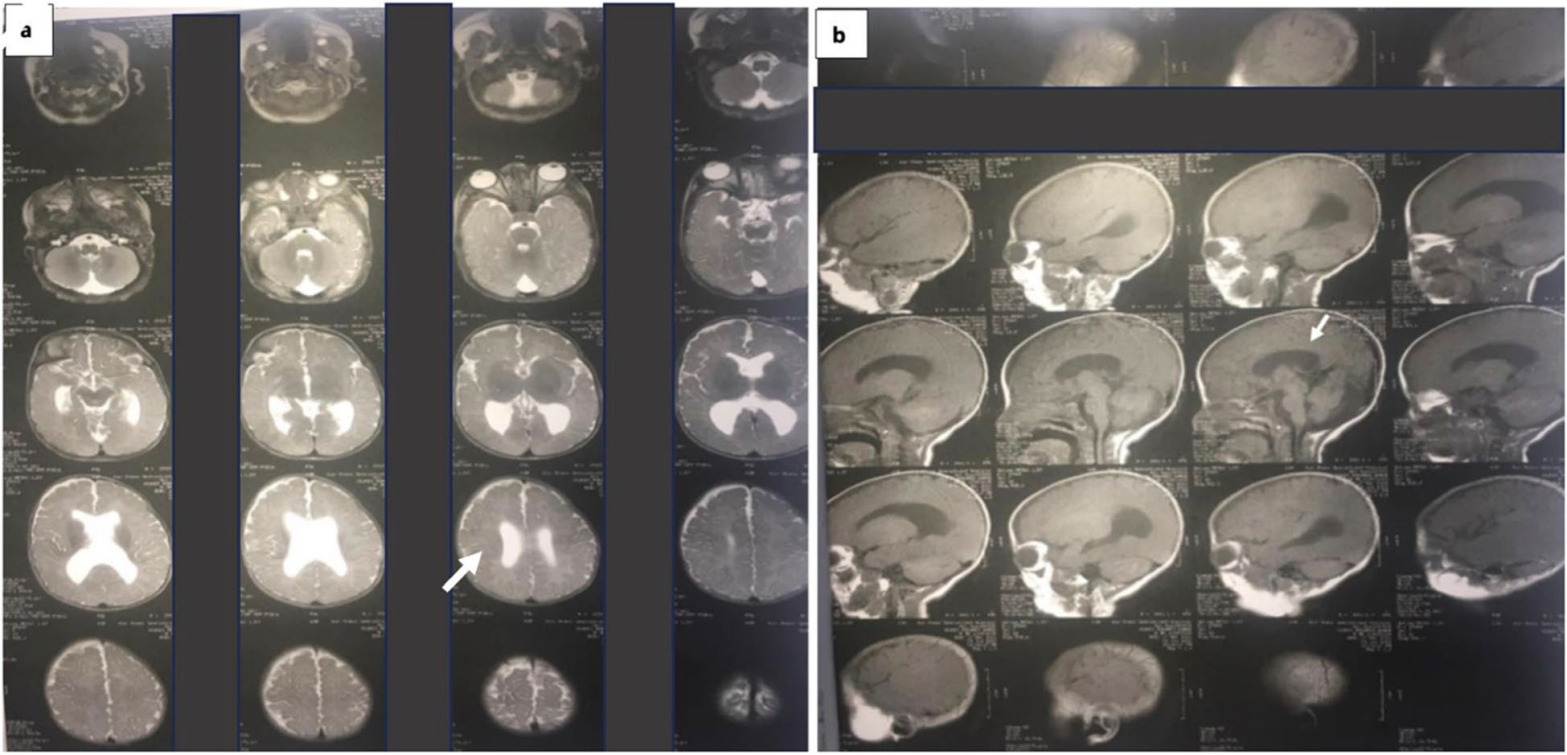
Brain MRI of patient 5 displayed agenesis of the corpus callosum. a. Axial T1-weighted brain MRI shows parallel lateral ventricles. b. The Sagittal T1-weighted MRI shows agenesis of the corpus callosum
